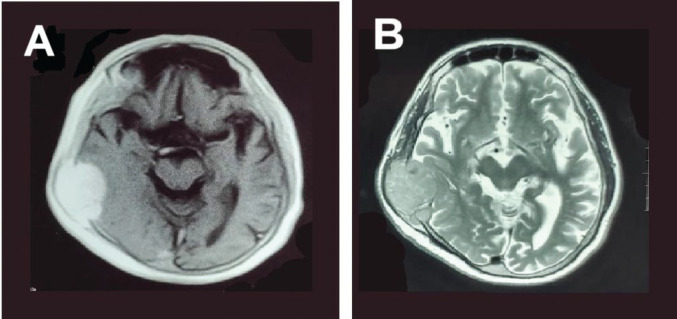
MRI brain scan with contrast, axial slices (a) T1-weighted and (b) T2-weighted images showing a homogenous well defined, isointense lesion in the right temporoparietal region causing compression and destruction of the cortex of the temporal and parietal bone.
